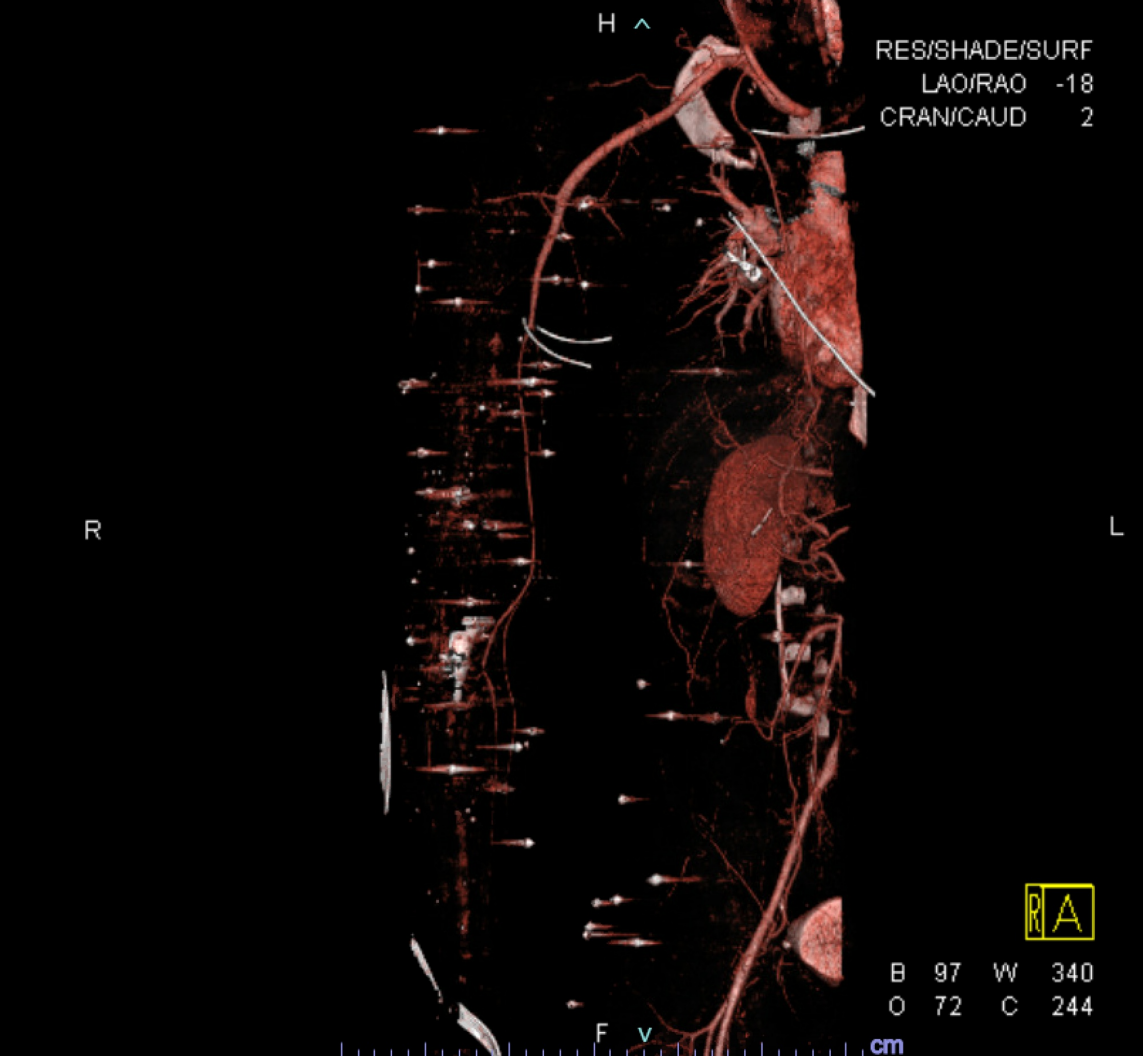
CTA 3D reformat right upper extremity Image shows pellets with streak artifacts of the right upper extremity and right abdomen
